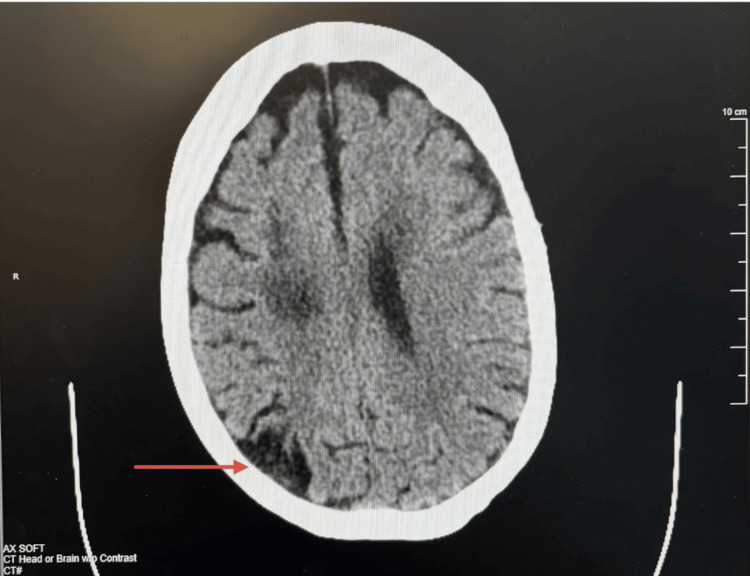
CT of the head/brain (noncontrast) showing chronic right parieto-occipital infarct and probable chronic basal ganglia and left thalamic infarcts (red arrow displays right parieto-occipital infarct)
